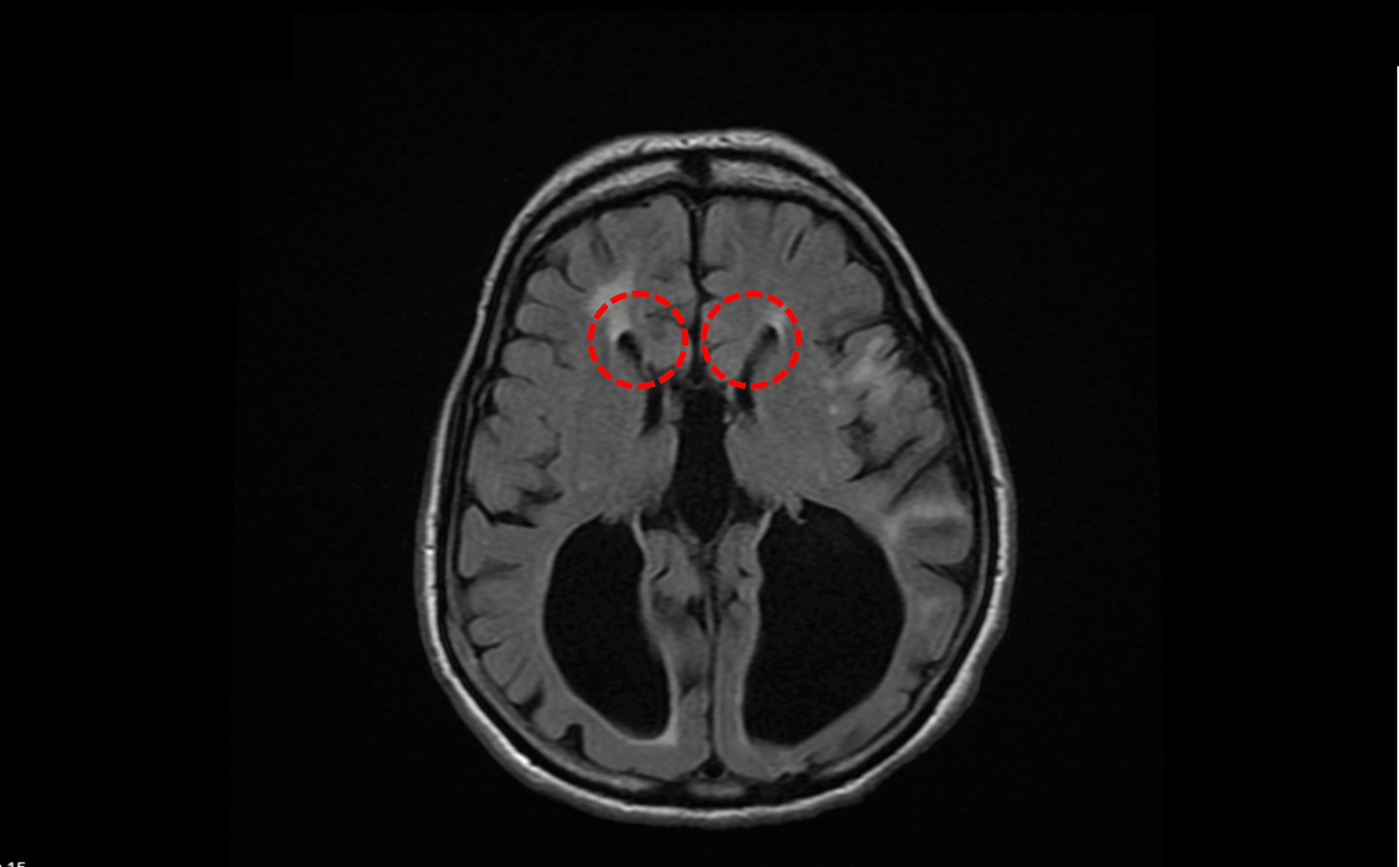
Coronal view of MRI brain showing a "moose head" appearance and enlargement of the lateral ventricles (red circle) due to agenesis of the corpus callosum MRI - Magnetic Resonance Imaging
